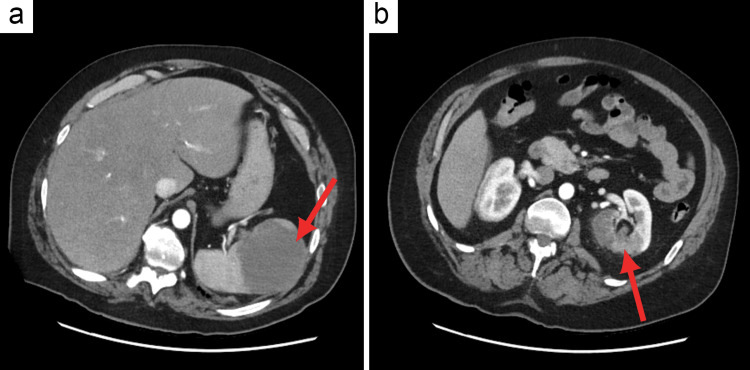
Computed tomography angiography of the patient’s abdomen. Repeat computed tomography angiography of the chest, abdomen, pelvis, and run-off confirming the presence of additional infarcted sites (red arrow), suggesting a multi-organ thromboembolic involvement. (a) Splenic infarction. (b) Left renal infarction.
